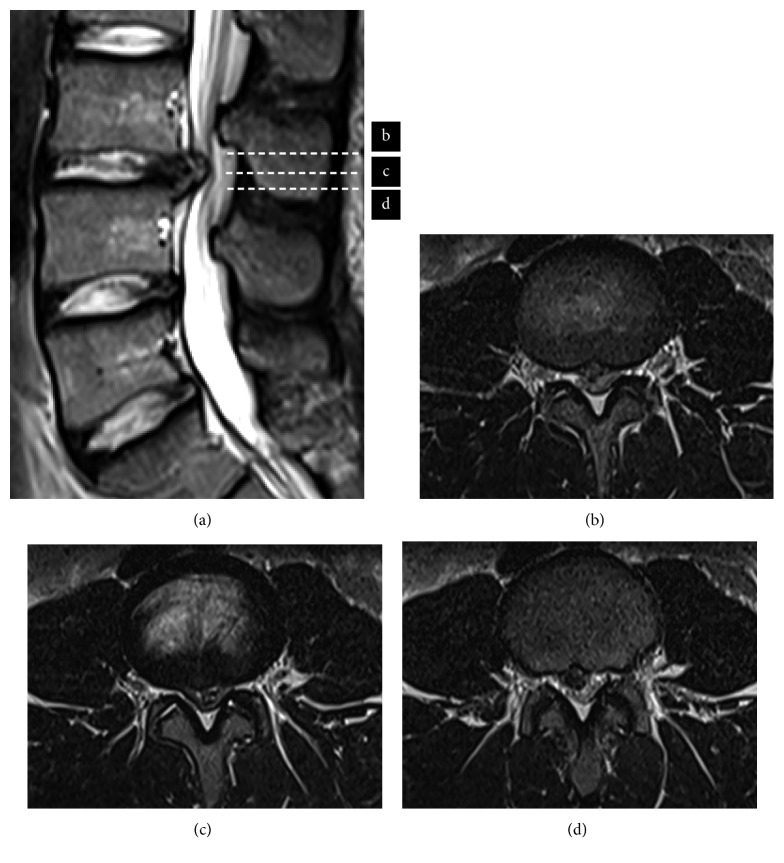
Preoperative sagittal T2-weighted MRI from sagittal view showed disc herniation at L3/4 disc level (a), and MRI from axial views showed left side huge herniation occupying the spinal canal (b–d).
